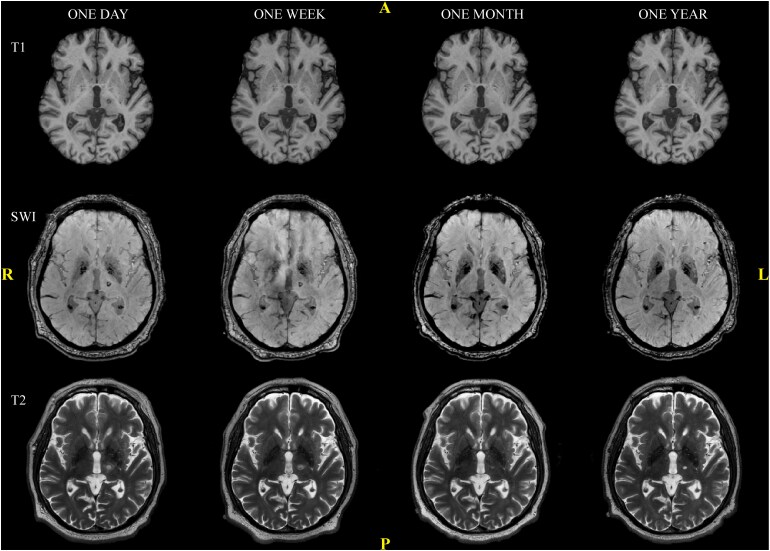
Lesion evaluation. Post-operative MRI scans of a patient acquired along follow-up. Rows, top to bottom: T1, SWI, and T2 weighted. Columns, left to right: one day, week, month, and a year after the procedure.
